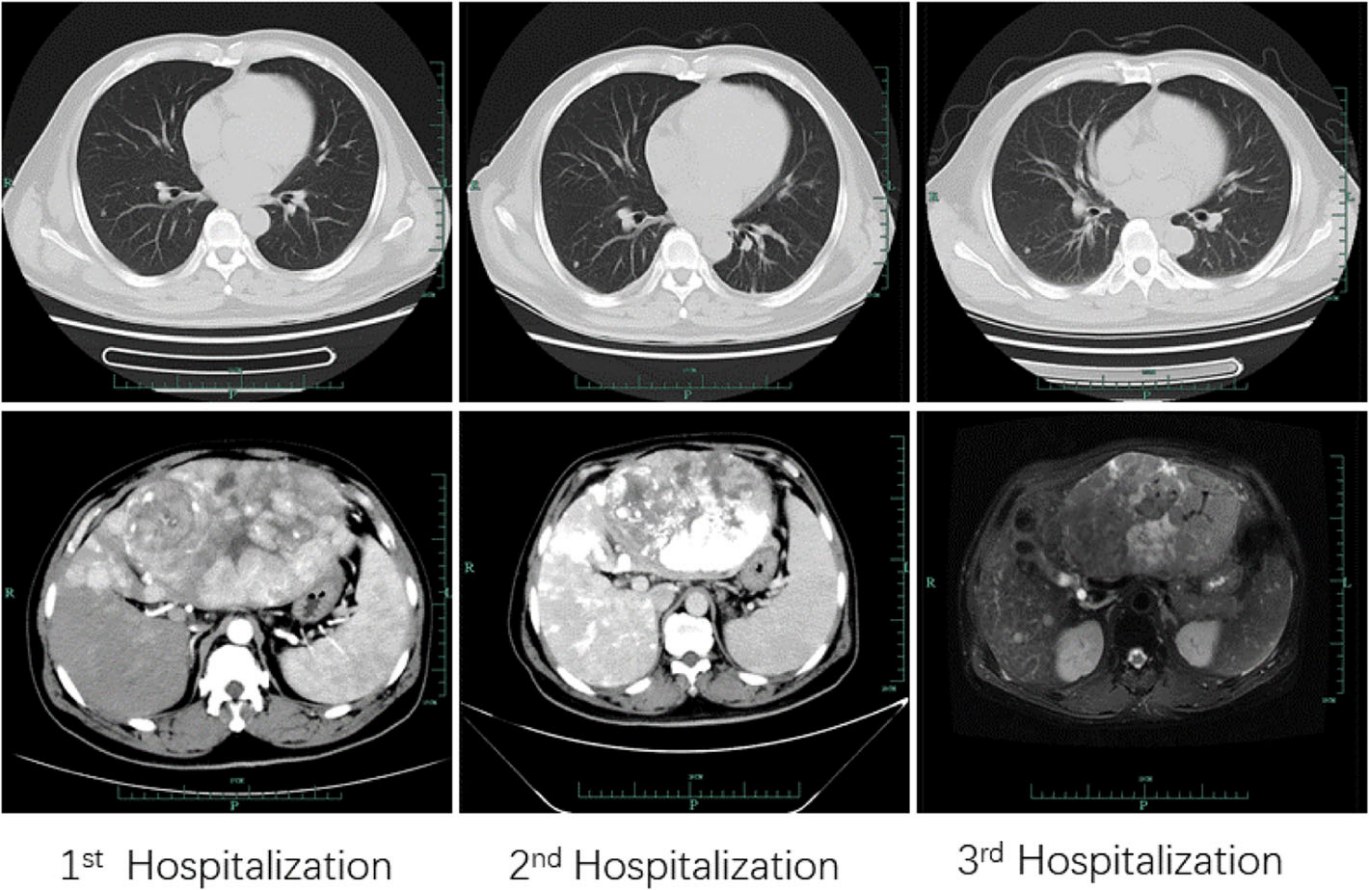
Imageological examination of three times of the hospitalization. The first row of chest CT were taken on September 19th, October 16th, December 2nd, respectively. The second row of liver CT or MRI were taken on September 20th, October 23th, December 2nd, respectively. The chest CT showed multiple subpleural nodules in lower lobe of both lungs. The enhanced CT or MRI showed the formation of left massive hepatocellular carcinoma before or after TACE therapies
